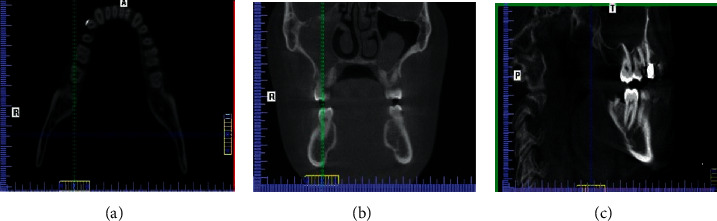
Cone-beam computed tomography images. (a) Axial view. (b) Coronal view. (c) Sagittal view.
